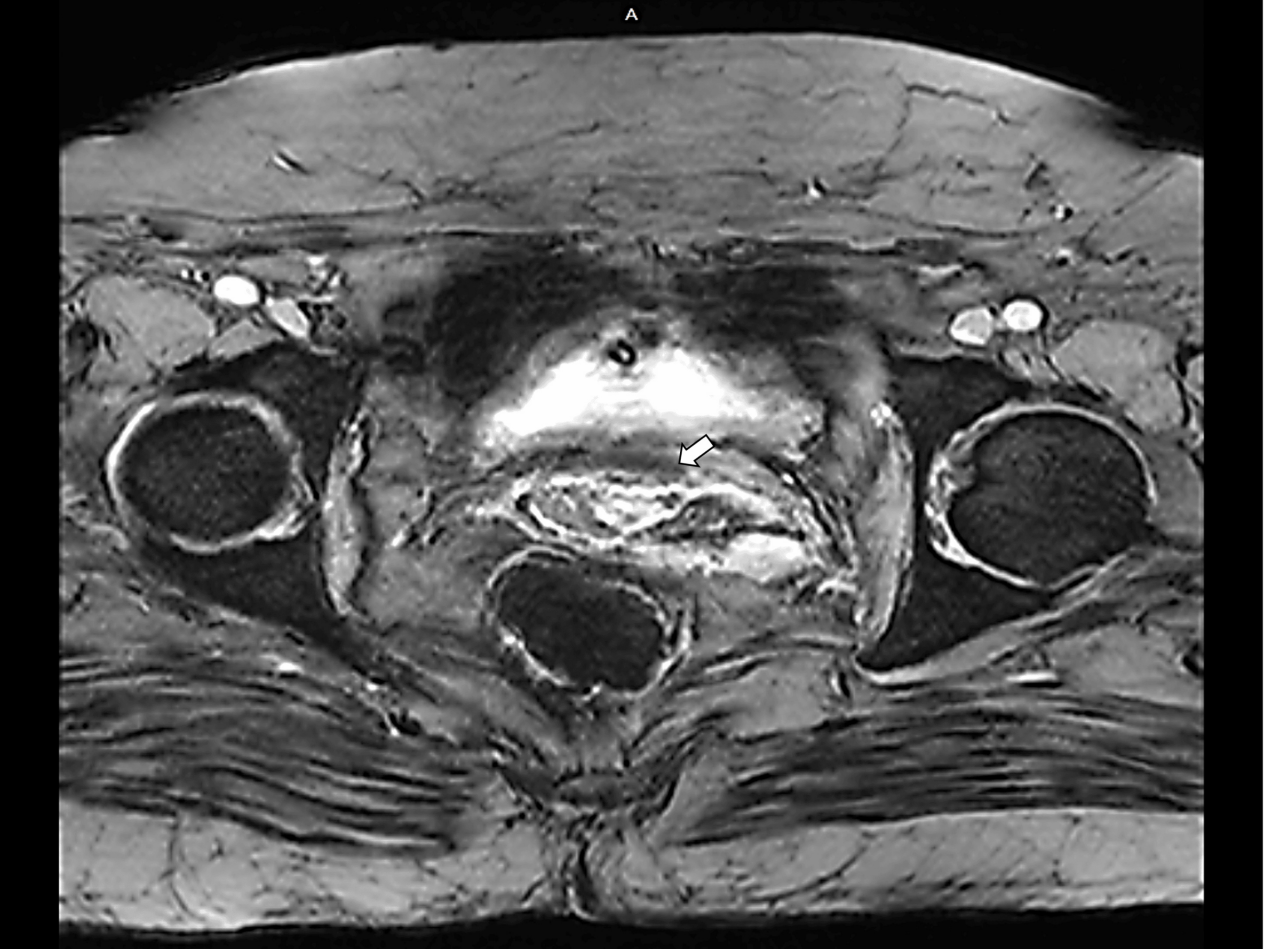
T2-weighted gradient recalled echo (GRE) axial image White arrow showing a well-defined T2 hyperintense lesion in the anterior cervix with preserved fat planes between the lesion and the urinary bladder. No regional lymphadenopathy is seen, and diffuse thickening of the vaginal wall is noted.
